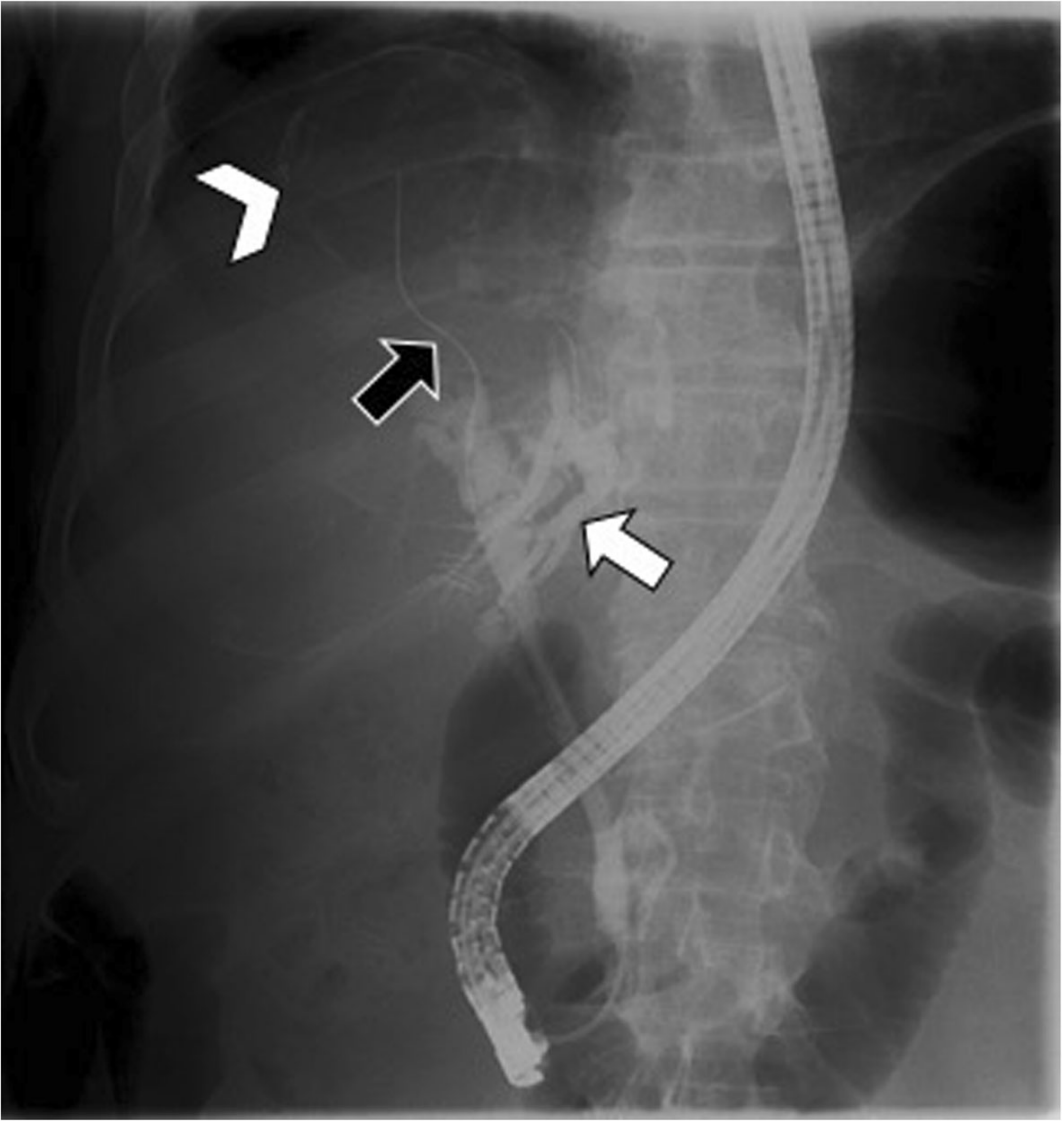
A 72-year-old man with communicating rupture. Radiographic image performed during ERCP demonstrates a linear calcified opacity (arrowhead)—representing a hepatic hydatid cyst—and intrahepatic bile duct dilatation (white arrow). The catheter (black arrow) introduced in biliary tree enters directly into the cyst, documenting the cysto-biliary fistula
